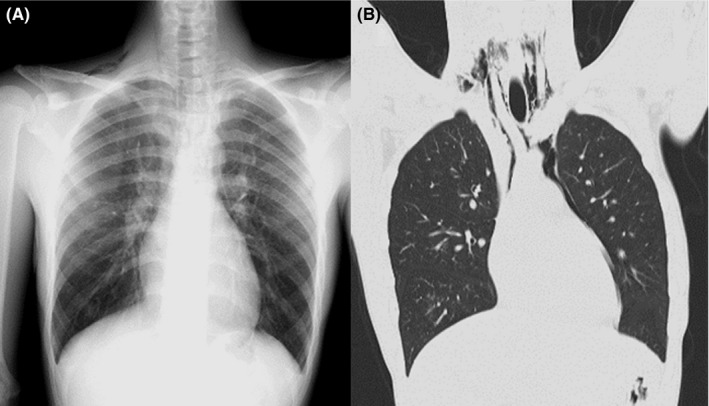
(A) Chest X‐ray showing emphysema above the right clavicle and in the mediastinum. (B) Chest computed tomography shows emphysema in the mediastinum and behind the right clavicle
